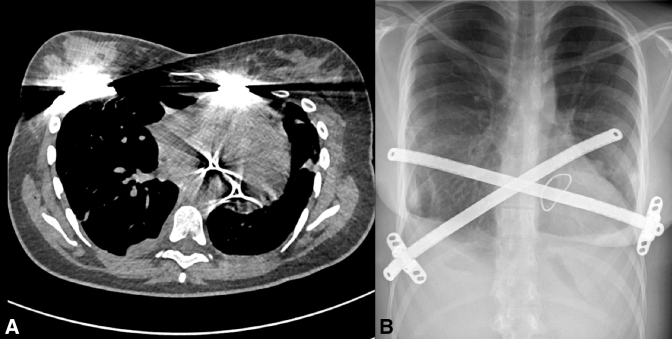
A, Postoperative computed tomography findings: The modified cross-bar Nuss repair in Grand Canyon–type PE showed improvement in the sternal depression (Haller index, 2.06). B, Postoperative chest radiograph after cross-bar technique.
